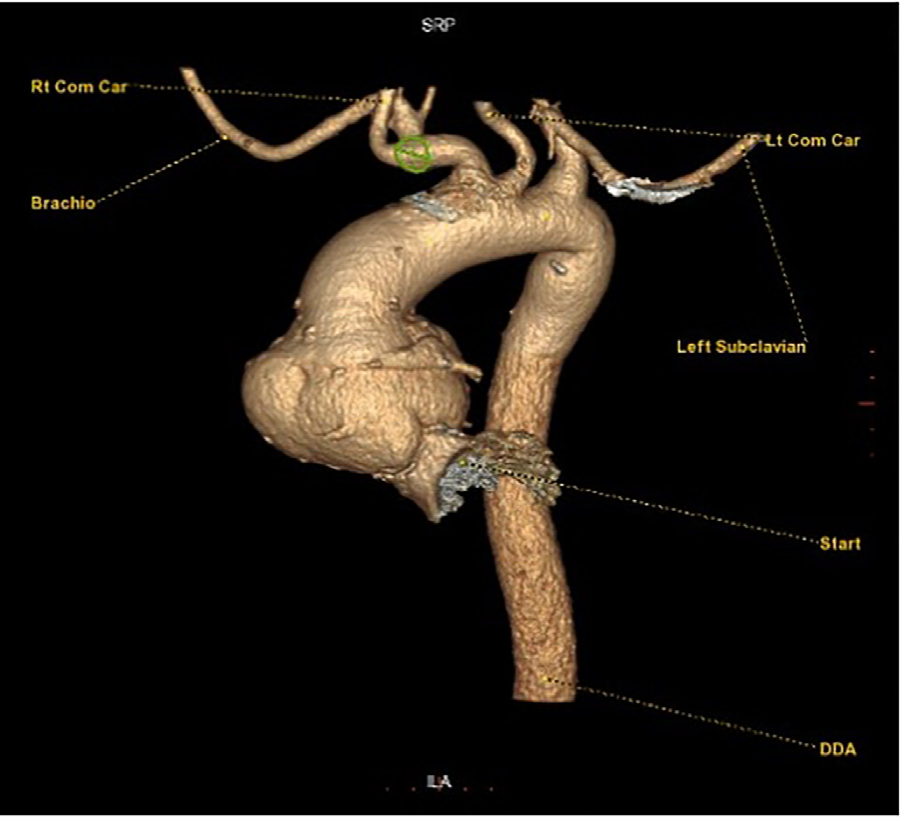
A 3D (three dimension) VR (volume rendering) image created on the preoperatory CT scan showing the localization of the aneurysm at the aortic root level.
